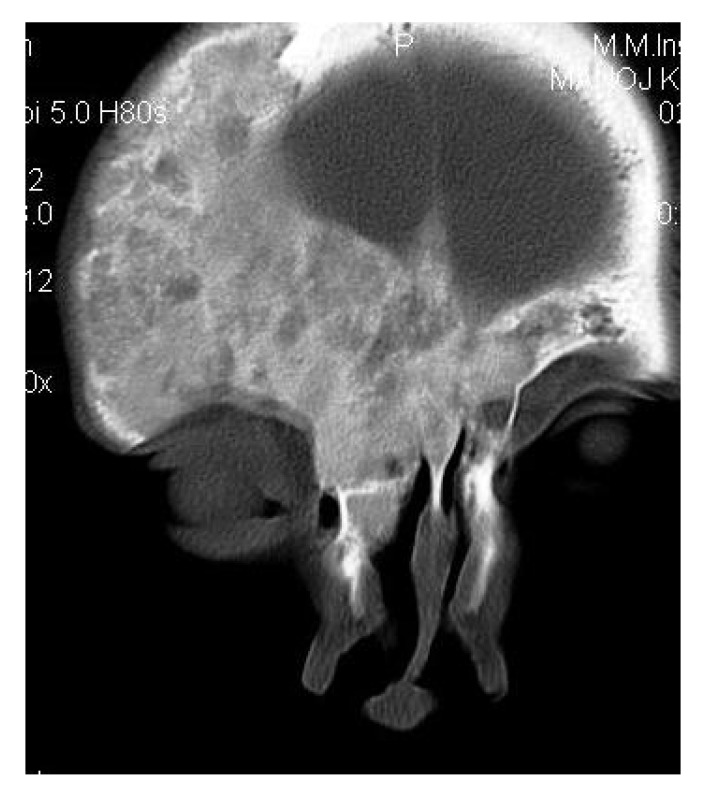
Coronal CT section revealing the lesion crossing the midline in frontal region with involvement of Crista Gali.
